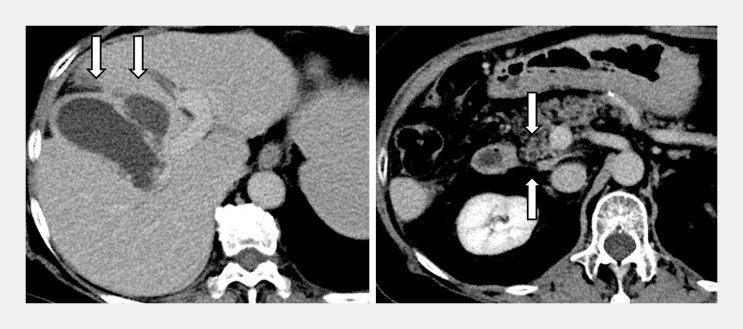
Portal phase of contrast-enhanced computed tomography (CT) showing an enlarged gall bladder and a gall bladder-surrounding abscess (left). The wall thickening of the distal bile duct is observed, but common bile duct stones cannot be identified on CT (right).
